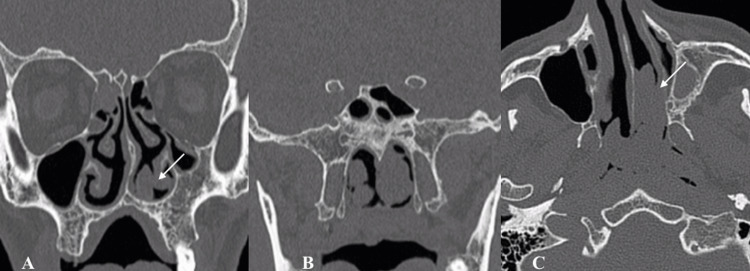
Inferior turbinate IP CT sections showing an IP (indicated with an arrow) in a 23-year-old male patient. A: Coronal CT scan showing a soft tissue mass originating from the left inferior turbinate, associated with bony remodeling (arrow). B: Posterior coronal CT section demonstrating ethmoid sinus involvement with adjacent bone thinning. C: Axial CT section of the same mass, with evidence of bone remodeling without aggressive destruction (arrow) CT: computed tomography; IP: inverted papilloma
